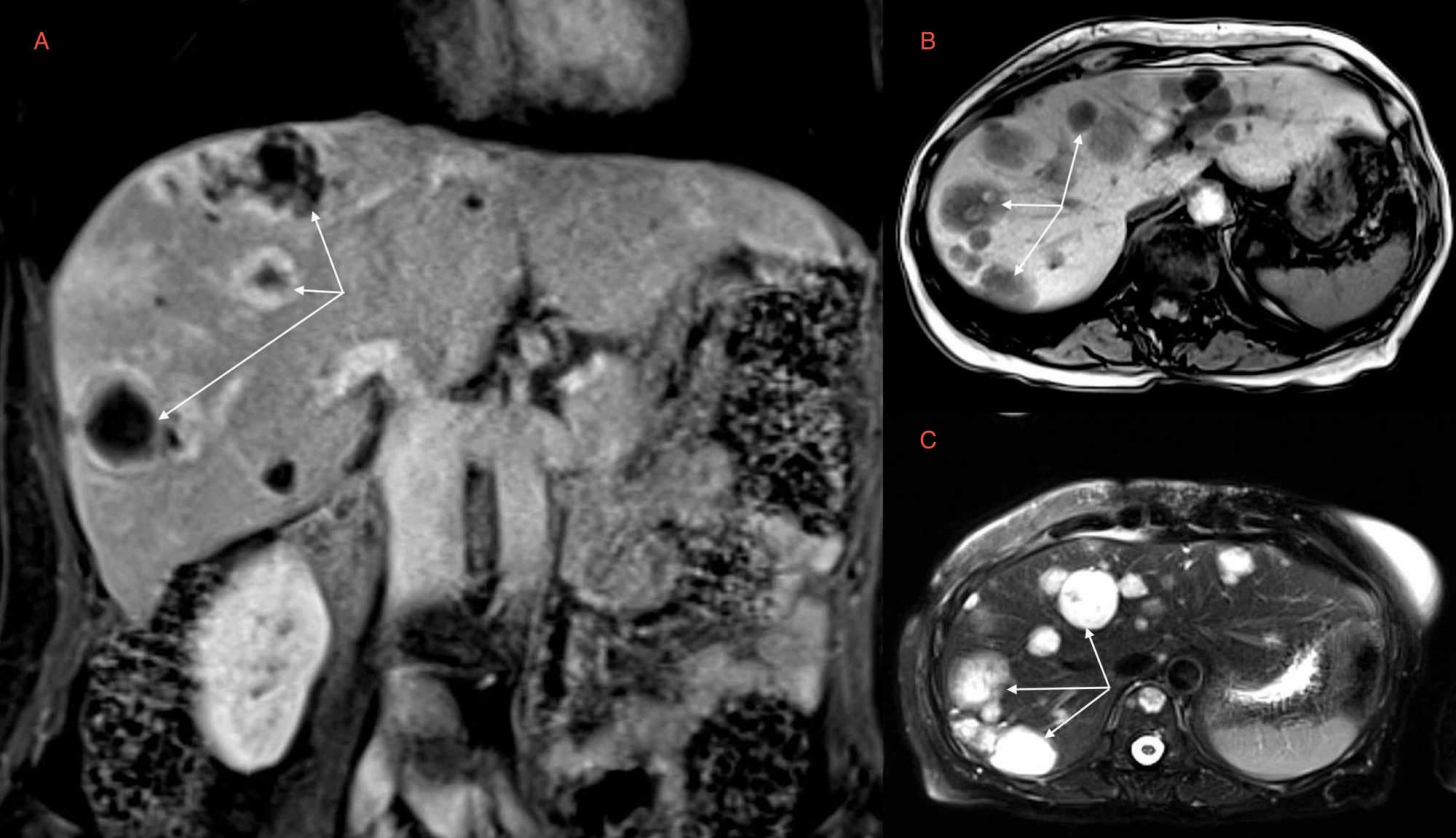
Abdominal Magnetic Resonance Imaging (A) T1 weighted coronal section, (B) T1 weighted axial section, and (C) T2 weighted axial section of abdominal magnetic resonance imaging with arrows highlighting multiple enhancing lesions that were concerning for recurrence of malignancy
